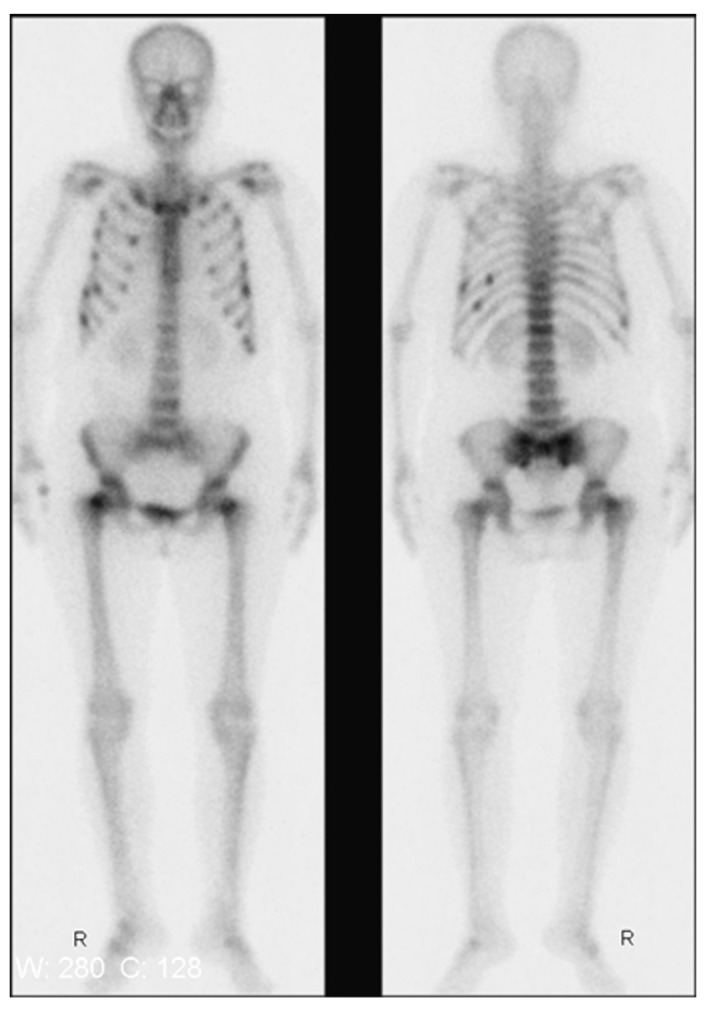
Whole-body bone imaging emission computed tomography. Whole-body positron-emission tomography CT (PET-CT) examination showed that several ribs and double femoral neck (incompletely) had discontinuity and numerous old fracture lines (loose lines) were present, in addition to mild T12 compression changes. Bilateral hip joint swelling was accompanied by an increase in FDG metabolism and these regions were classified as inflammatory hip lesions.
